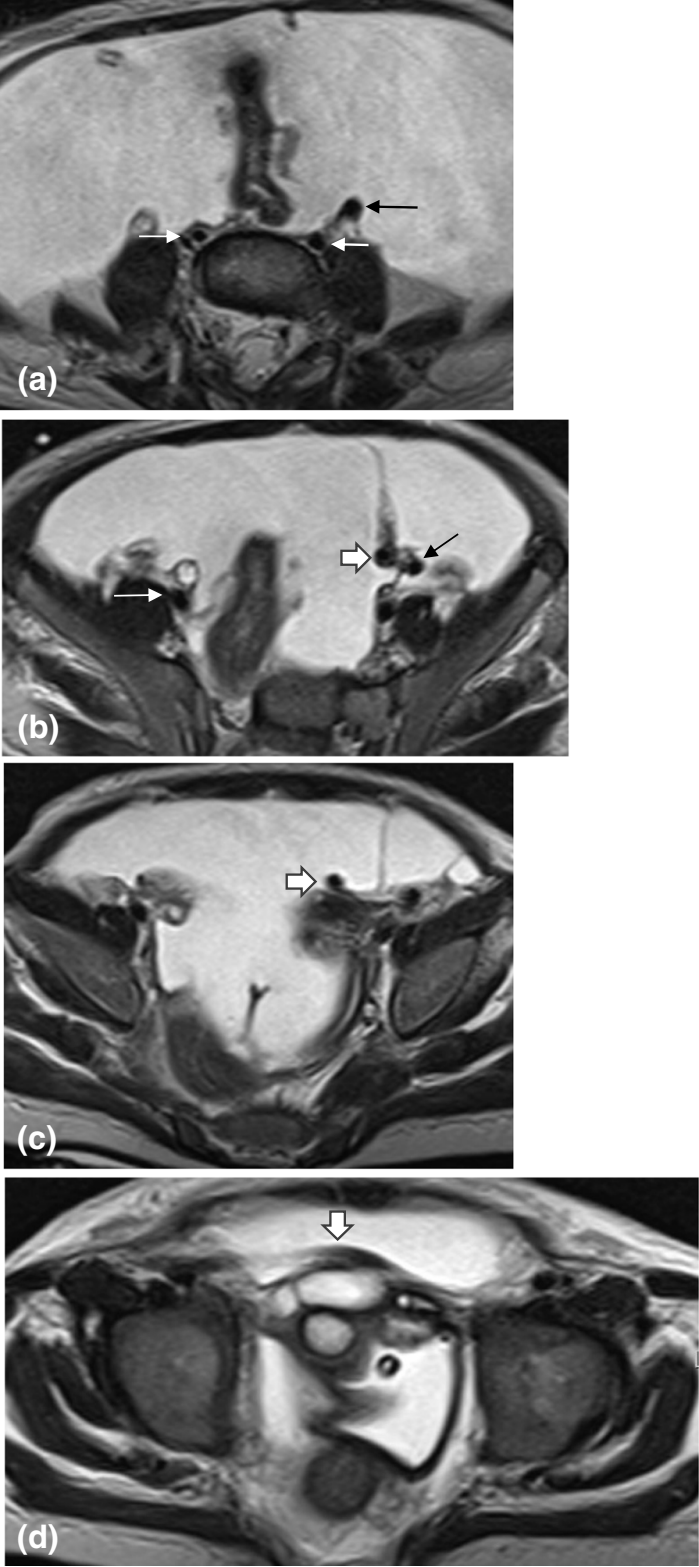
a Axial T2 MRI of the abdomen at the level of the common iliac vessels, showing bilateral common iliac veins (white arrows). The left common iliac artery is present (black arrow). However, the right is absent. b, c and d More inferior axial T2 images at the level of the external iliac vessels showing the right external iliac vein (white thin arrow), without an accompanying artery. The left external iliac artery (black arrow), gives rise to a large vessel (thick white arrow) that crosses to the right side and passes superficially just posterior to the anterior abdominal wall, anterior the insertion of the distal ureters. This artery then continued as the right common femoral artery. The internal iliac vessels were absent bilaterally
